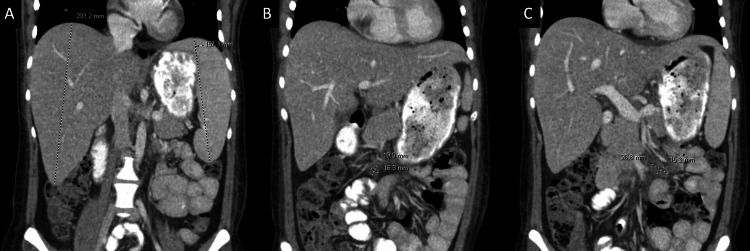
Case 2: computed tomography showing mesenteric adenopathy and hepatosplenomegaly (indicated) Moderate lymphadenopathy within the small bowel mesentery. Coronal intravenous contrast material–enhanced CT images show (A) hepatosplenomegaly (dashed lines) and (B and C) prominent mesenteric lymph nodes (dashed crosses) in the superior mesenteric artery/superior mesenteric vein mesenteric distribution.
